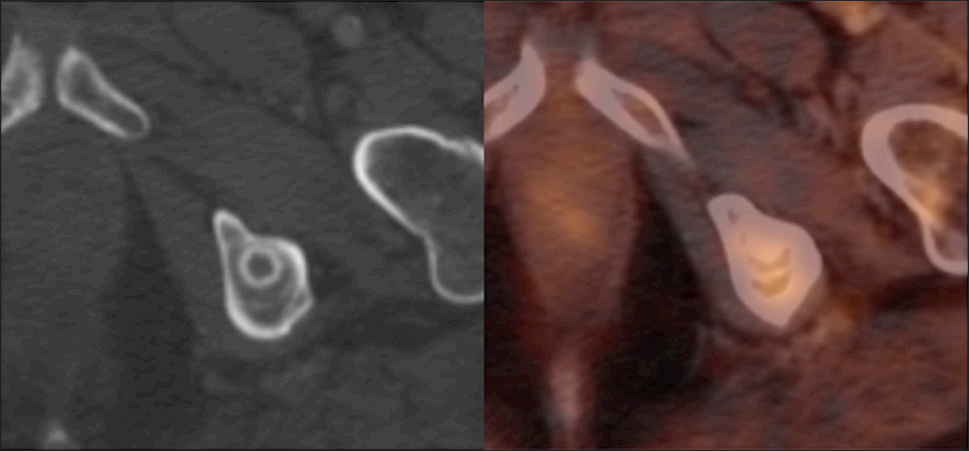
There is high uptake of FDG tracer on PET CT in this metastasis from a malignant peripheral nerve sheath tumor.
